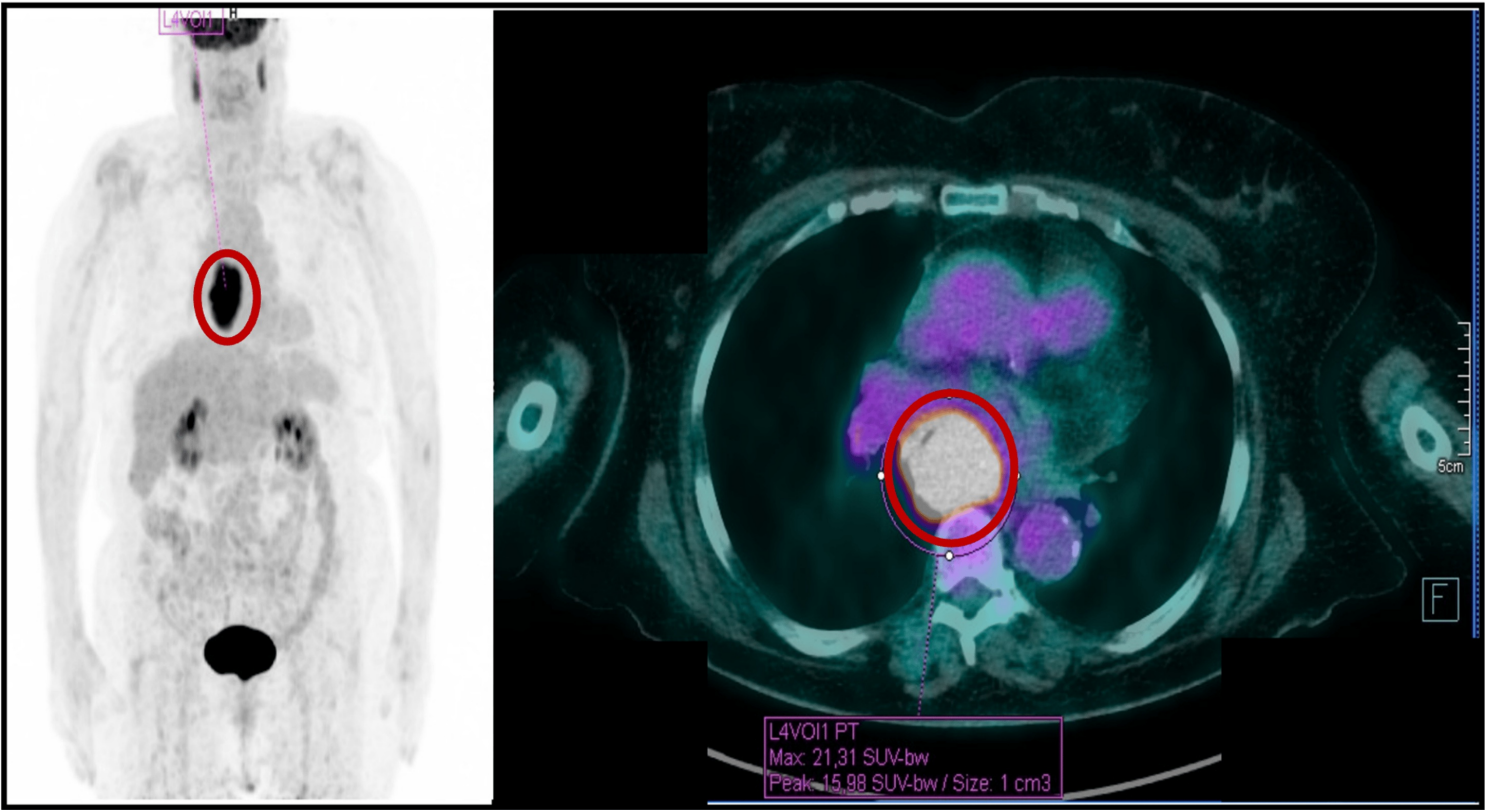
A PET-CT scan showing intense uptake in the lesion located in the middle/distal third of the esophagus (red circle), with an SUVmax of 21.3 PET-CT, positron emission tomography/computed tomography; SUVmax, maximum standardized uptake value
